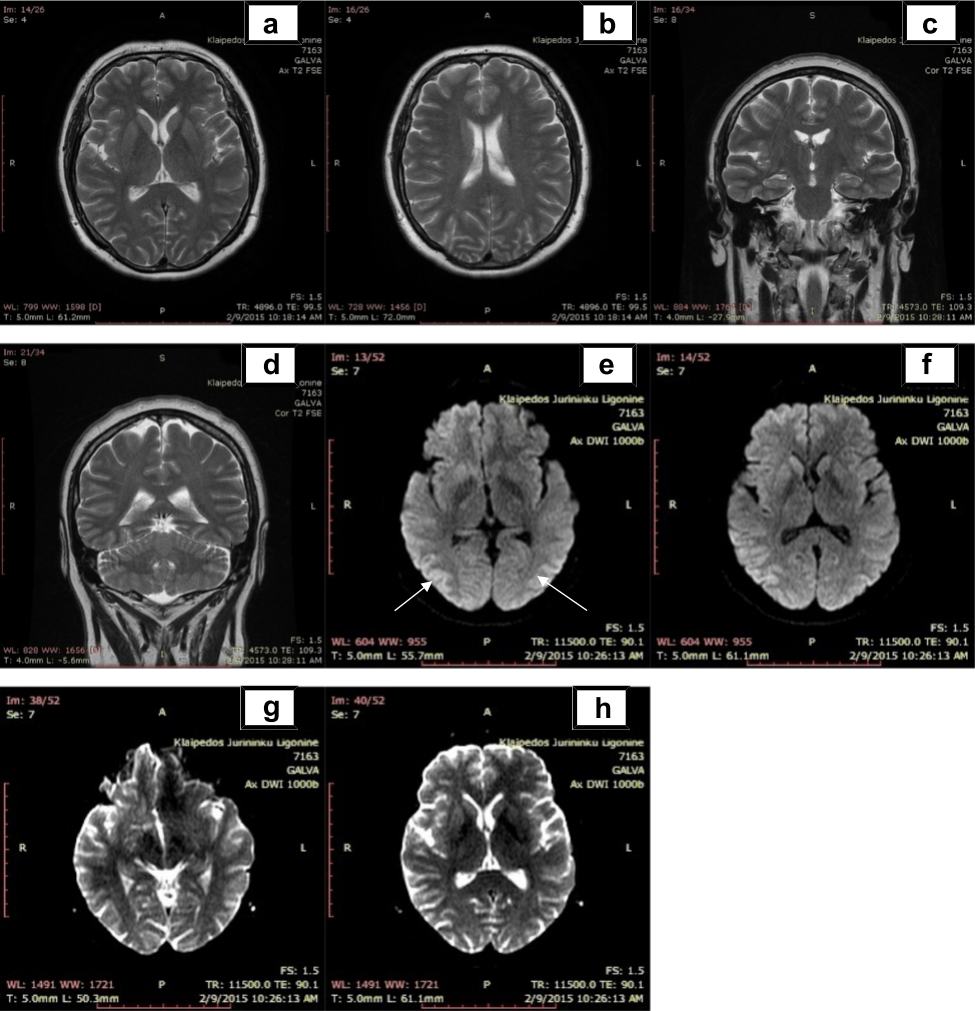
Brain magnetic resonance images at week 2. Legend: the primary evaluation – no significant changes. Retrospective reevaluation - slight increase in signal intensity in the occipital cortex DW sequence (e, f), without pathological lesions in the other sequences (a, b, c, d, g and h)
